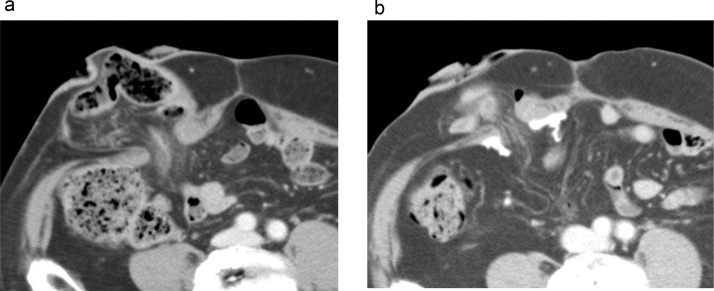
CT images of case 1. a. Preoperative abdominal CT showed herniation of the ileal loop into the subcutaneous space, compatible with parastomal hernia. b: CT image 2 years after the repair demonstrated no recurrence of parastomal hernia. There was no sign of mesh shrinkage or bowel obstruction.
